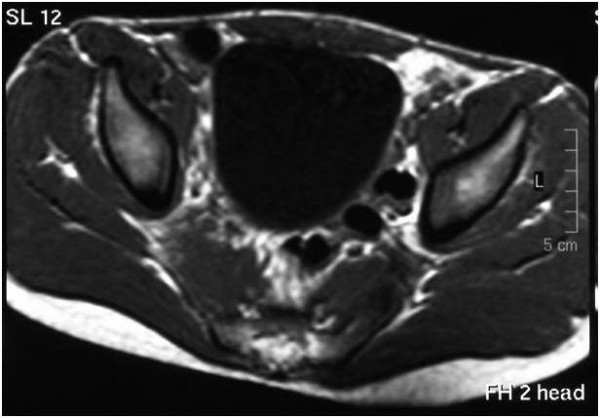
Axial spin-echo T1-weighted image; MRI scan taken at the time of antibiotic treatment initiation. The right piriformis muscle is enlarged. The surrounding fat planes are intact.
